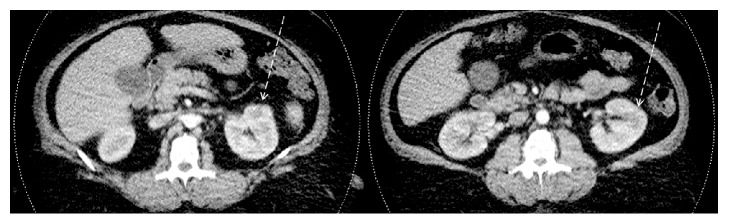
Findings. Abdomen CT scan with intravenous contrast after finishing 4 weeks of intravenous meropenem. It showed nearly complete resolution of the previously described abscesses with no perinephric fat stranding or collection.
